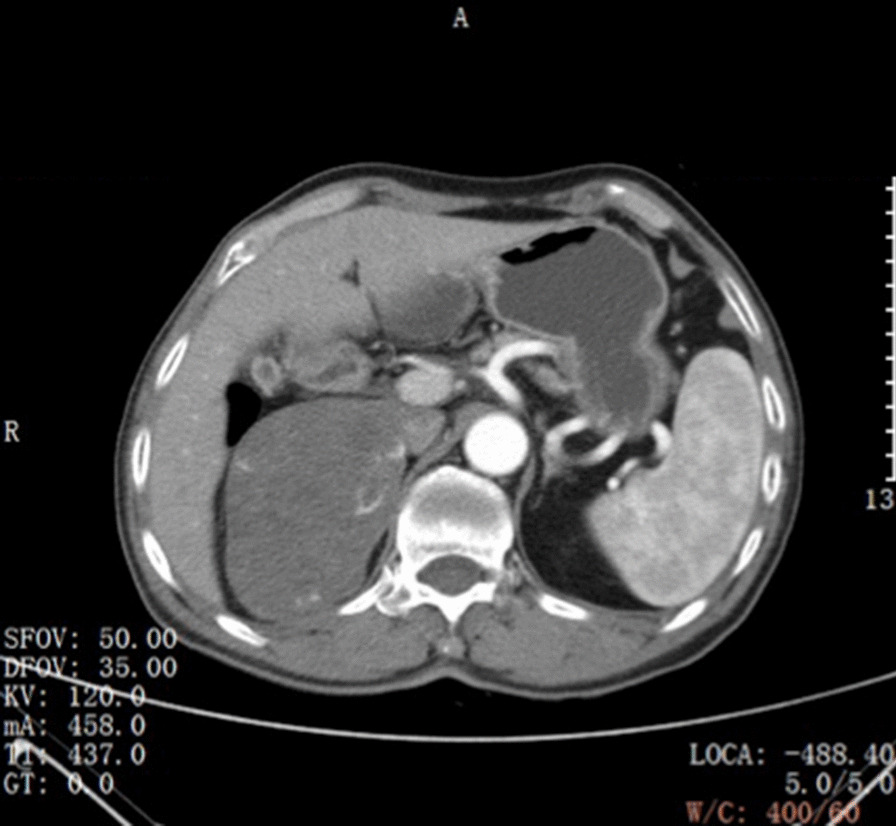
Contrast-enhanced CT images obtained in the arterial phase, 3 min after iodinated contrast administration
